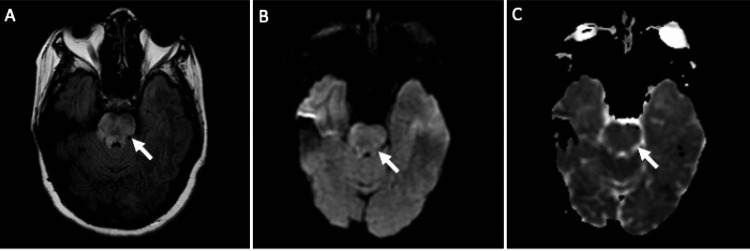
MRI scans from the second presentation. Arrows in each panel indicate the pontine region of interest. A) Axial T2 fluid attenuated inversion recovery imaging (B) Axial diffusion weight imaging (C) Axial apparent diffusion coefficient imaging
